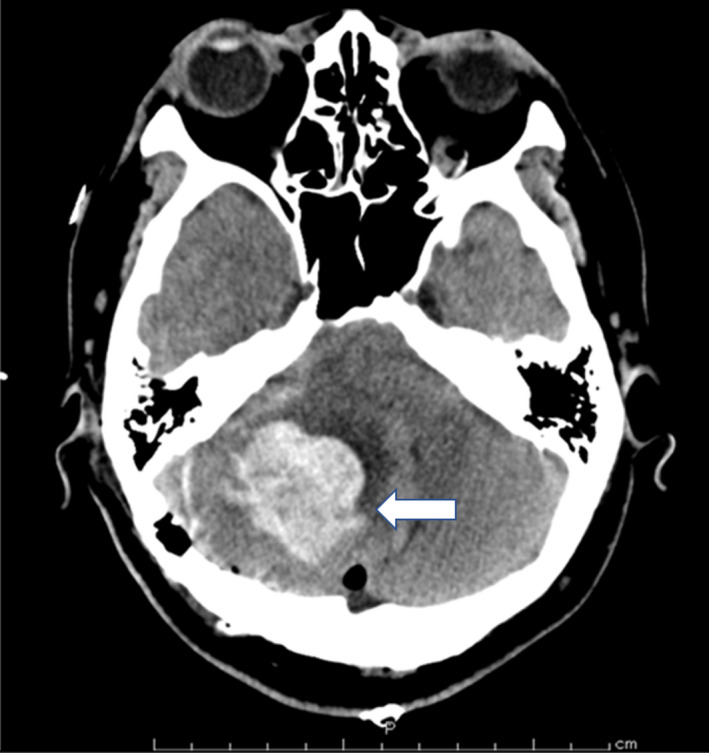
Postoperative brain computed tomography. Arrow shows cerebellar hemorrhage (30 × 37 mm)
